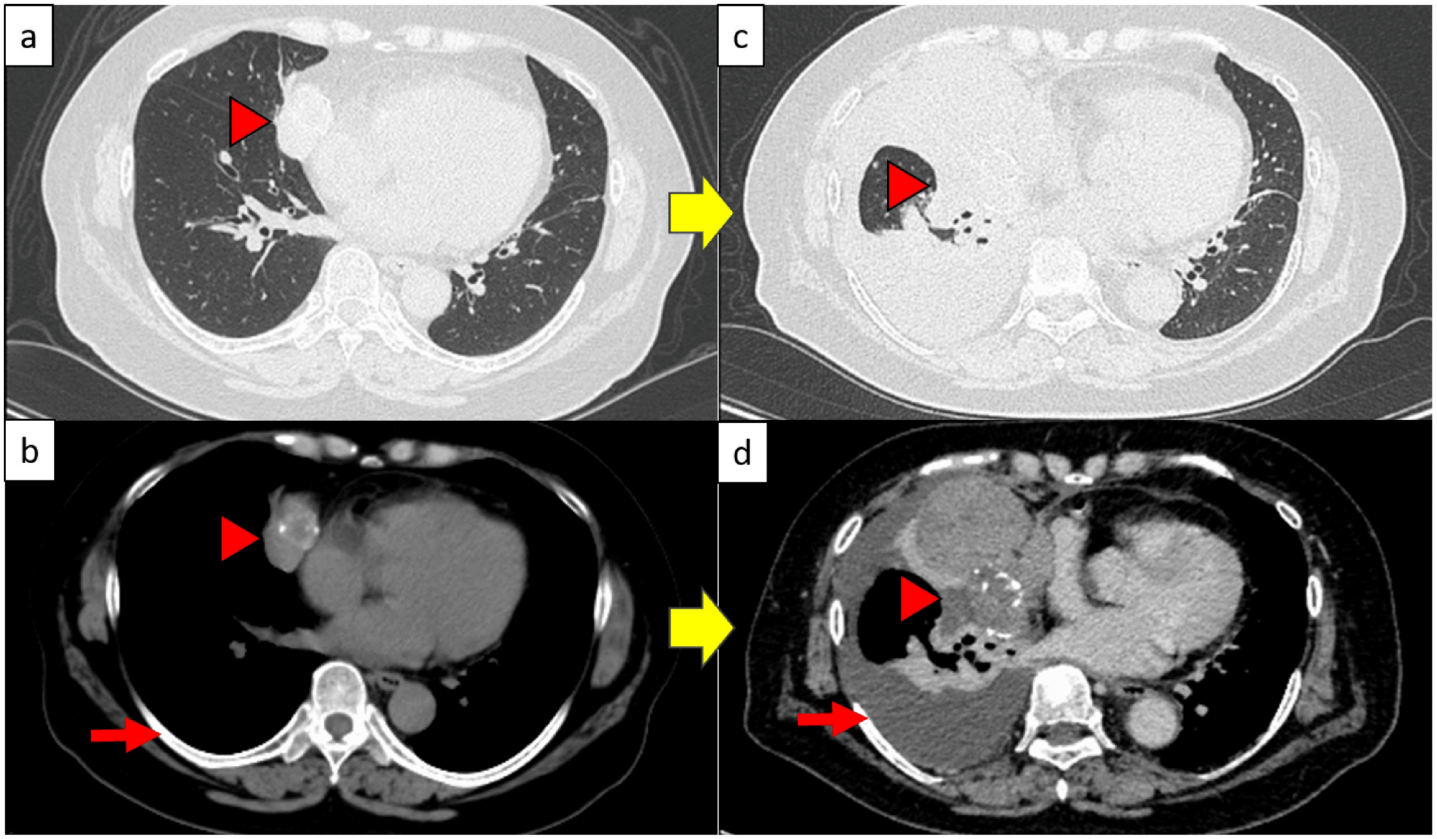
Chest computed tomography (CT) results The image findings in panels (a) and (b) comprise CT images taken 12 years earlier, and those in (c) and (d) are initial chest CT results. (a) A mass shadow was observed in the anterior mediastinum. No nodular shadows were observed due to pulmonary metastasis. ▶: Mass (b) The mass shadow in the anterior mediastinum from (a) measured 45 × 41 × 28 mm and was accompanied by calcification. ➡: No pleural effusion was observed. ▶: Mass (c) Tumor growth- and pleural effusion-related compressive atelectasis was observed. ▶: Compressive atelectasis (d) The tumor reached the size of 95 × 82 × 80 mm and right pleural effusion was noted. ➡: Pleural effusion was observed. ▶: Mass
